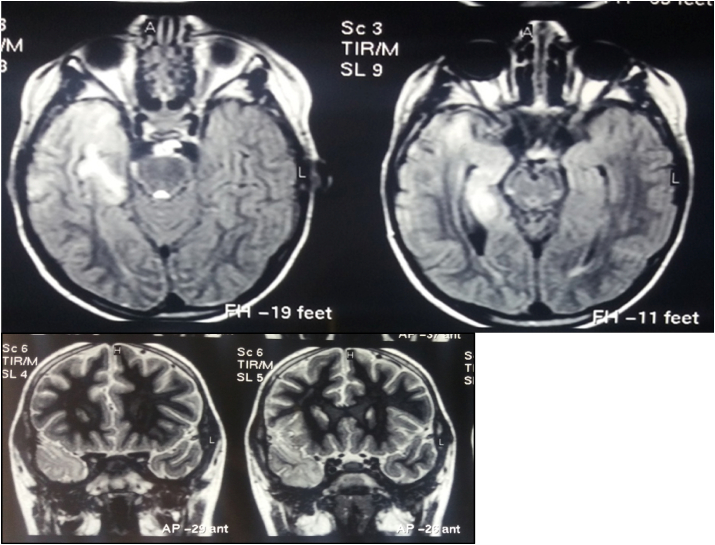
A: MRI Axial Flair T1, B: Coronal T2: Show high signal intensity area in right temporal lobe and Hippocampus.
